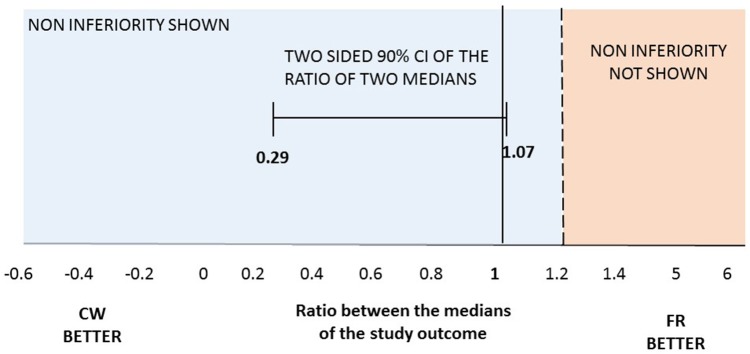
Two-sided 90%CI for the ratio between median number of cumulative Gd-enhancing lesions at MRI observed in the two regimens. Maximum ratio of 1.2 lesions was set for the one-tailed test with a significance level of 0.05. At month 12 median of the cumulative number of Gd-enhancing lesions at MRI was 1 (0–9) in CW vs. 3 (0–10) in FR patients.
